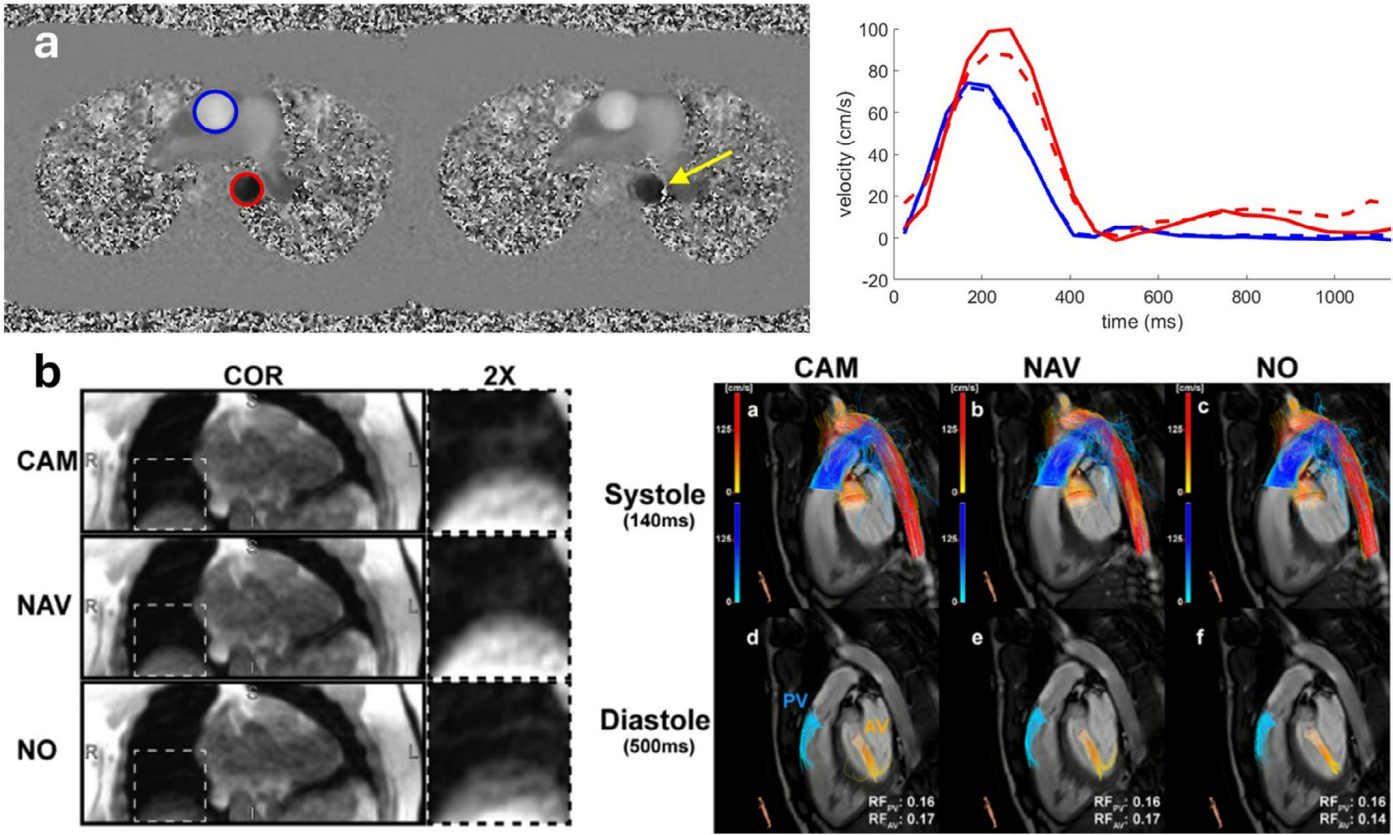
Motion in cardiac flow MRI. (a) Effects of misgating on 2D phase contrast MRI. Velocity images at peak systole for correct gating (left) and misgating (middle), in which 5 of the 16 heartbeats during acquisition were corrupted by arrhythmia, causing additional noise in the descending aorta (yellow arrow). The effects of this misgating (right) are substantial in the descending aorta (red), leading to incorrect velocity measurements (dashed line). (b) Example 4D flow magnitude coronal images using in‐bore camera‐based (CAM) gating, navigator‐based (NAV) gating, and no respiratory gating (NO). The liver‐lung interface (dotted box) shows improved delineation in both respiratory gating techniques. Systolic and diastolic streamlines (right) of CAM, NAV, and NO data sets. Slightly higher regurgitation fraction (RF) through the aortic valve (AV) was found after respiratory gating. PV: Pulmonary valve. Adapted from [20].
